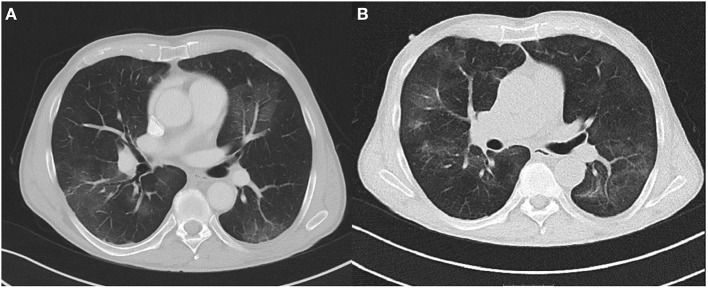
(A) Chest CT at day 5 showing bilateral peripheral ground-glass opacities. (B) Chest CT at day 17 showing opacities at different locations without significant reduction.
